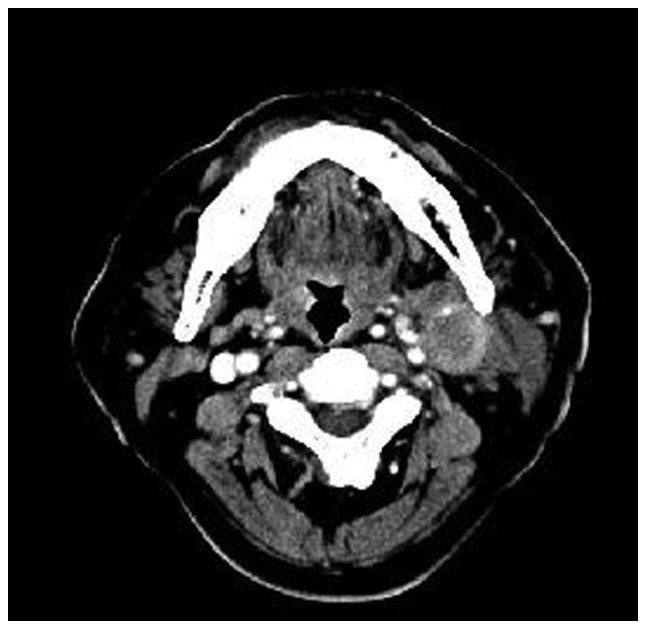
Axial, contrast-enhanced computed tomography shows a hypodense and cystic lesion with ring enhancement, located in the deep neck-space on the left side of the neck.
